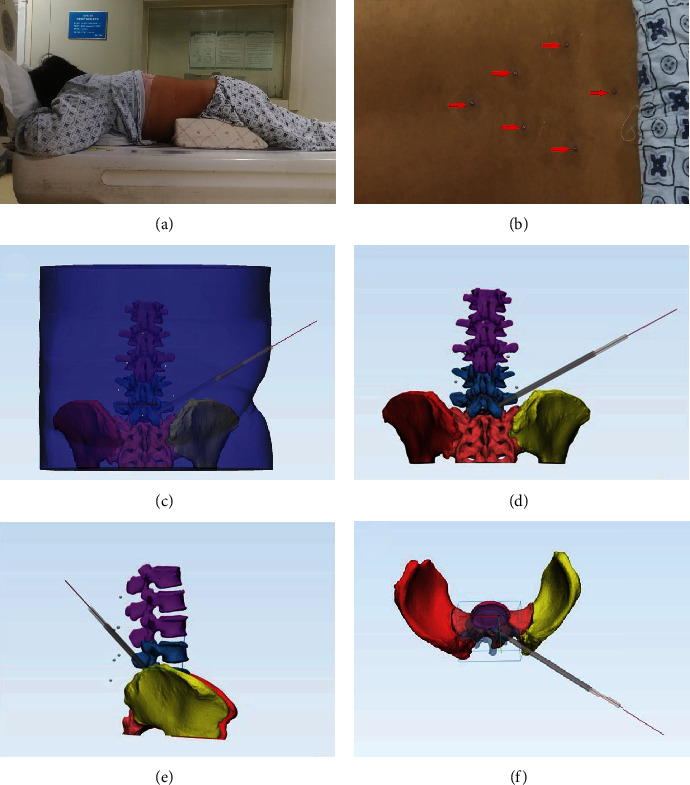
Computed tomography (CT) examination and reconstruction of the three-dimensional (3D) model. (a) Flexion position during lumbar CT examination in which the patient was positioned in the prone position on a hard sponge cushion. (b) External landmarks (red arrows) attached to the skin around the surgical segment during the CT examination; these landmarks were used for preliminary matching. (c–f) 3D model reconstructed from the CT data.
